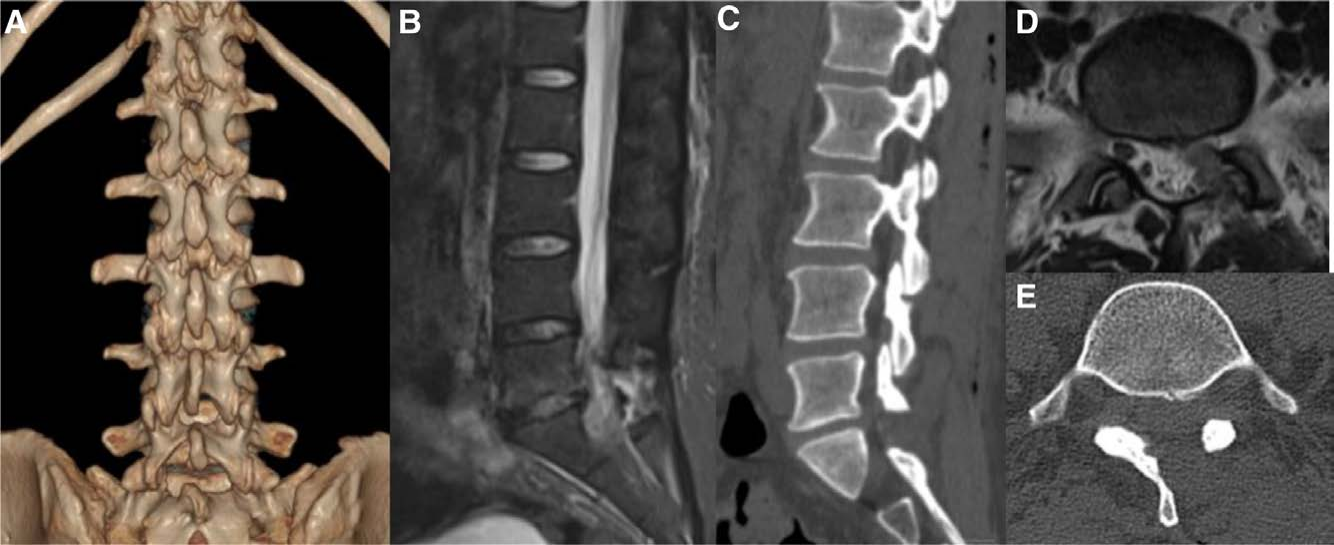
Patient postoperative radiologic images. (A) Three-dimensional reconstruction CT indicating the location of the L5-S1 laminectomy on the left side. (B–E) Postoperative MRI and CT images show that the protruding nucleus pulposus was successfully removed, and the spinal cord and nerve roots were completely decompressed.
